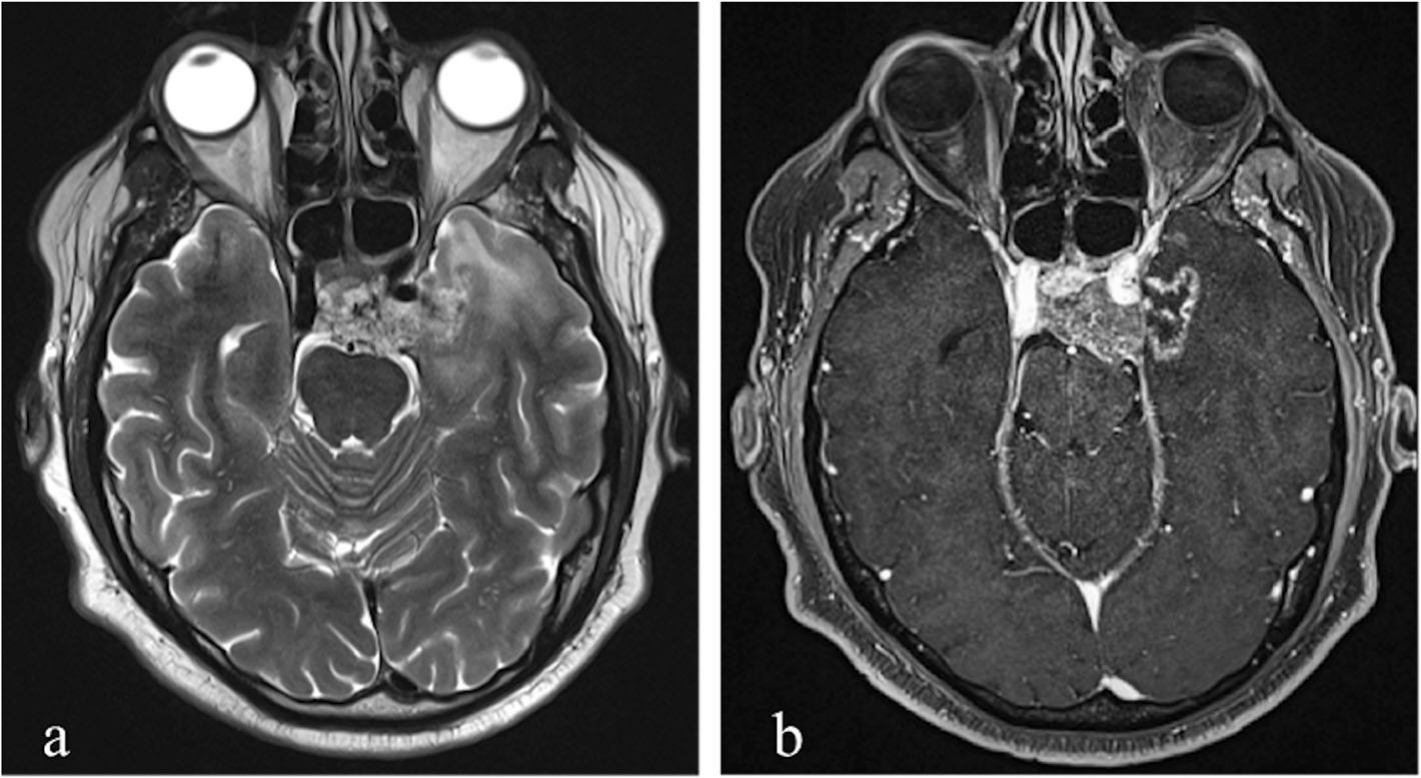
MRI performed 14 months after 74 Gy Proton-beam therapy in a patient who previously underwent subtotal surgical resection of clival chordoma. Axial T2-weighted image (a) shows hyperintensity involving subcortical white matter in left polar temporal region right adjacent to clival structures. Axial post-contrast T1-weighted image (b) reveals a focal non-enhancing portion related to necrotic tissue with a peripheral rim enhancement, as in grade 2 injury of LENT-SOMA scoring system
